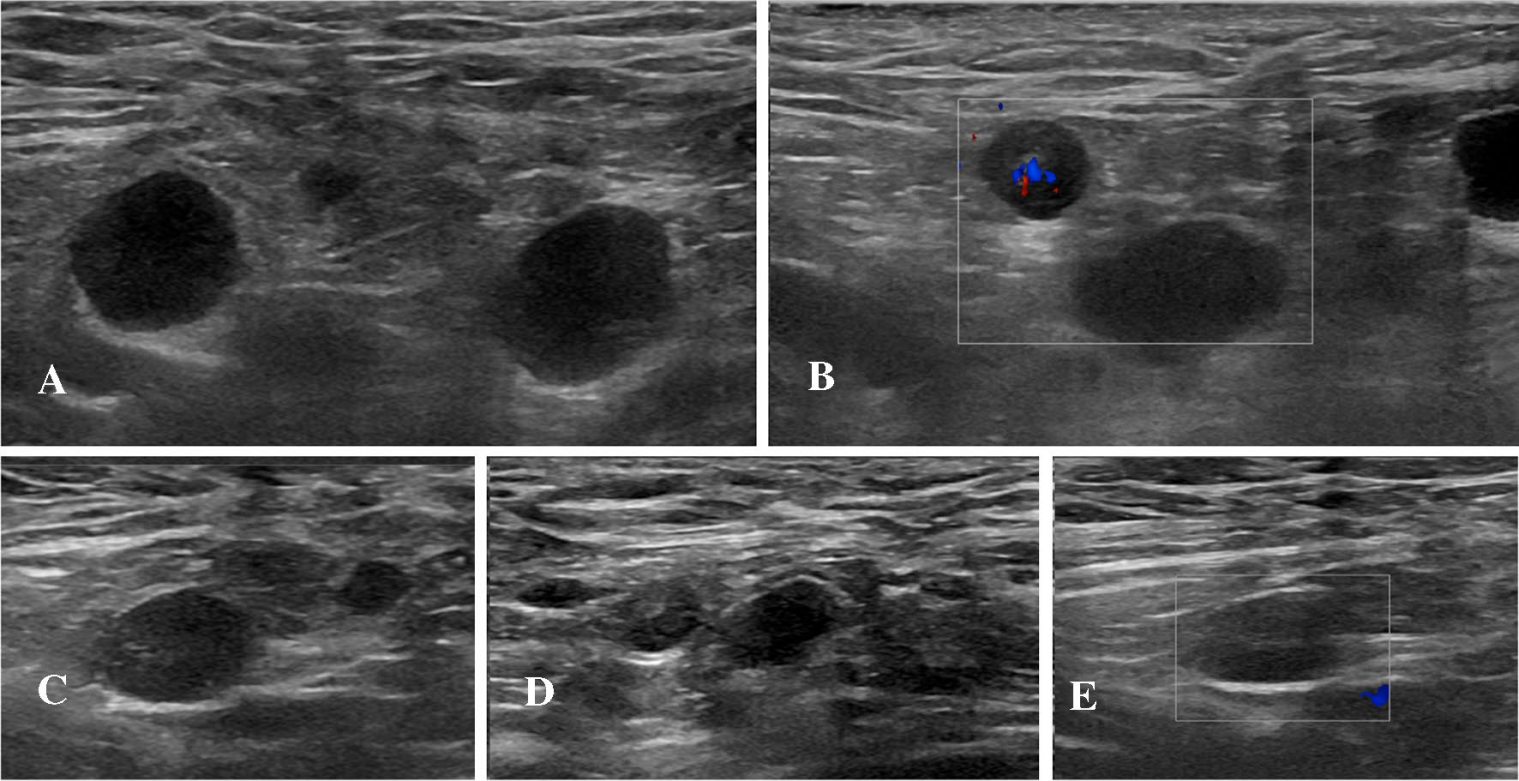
B-Mode (A) and color-Doppler (B) US examination performed 3 days after receiving the second vaccine dose. Multiple round (maximum diameter 11 × 10 mm) axillary lymph nodes with no fatty hilum and mild hilar vascularization were detected. B-Mode (C, D) and color-Doppler (E) US examination performed after 5 weeks. Only a reduction of lymph node size (maximum diameter 9 × 6 mm) was detectable.
